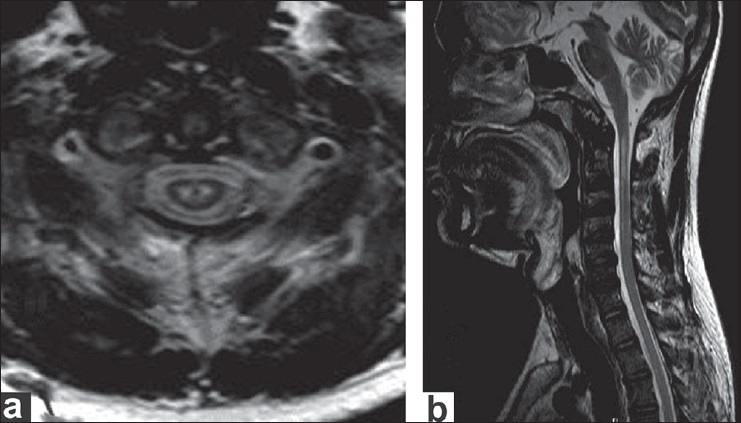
(a) Axial T2-weighted image at C1 vertebral level showing two focal T2 hyperintensities in the central part of spinal cord corresponding to the central grey matter of the cord. (b) Sagittal T2-weighted image showing ill-defined linear hyperintensity of the spinal cord extending between C1 and C4 vertebral body levels
